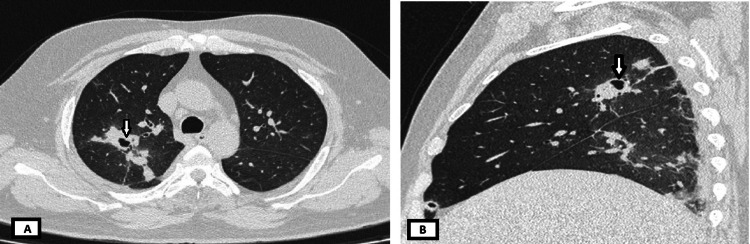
High-resolution computed tomography images of the thorax showing cavitary lesions with surrounding consolidation (A) Axial view. (B) Sagittal view
